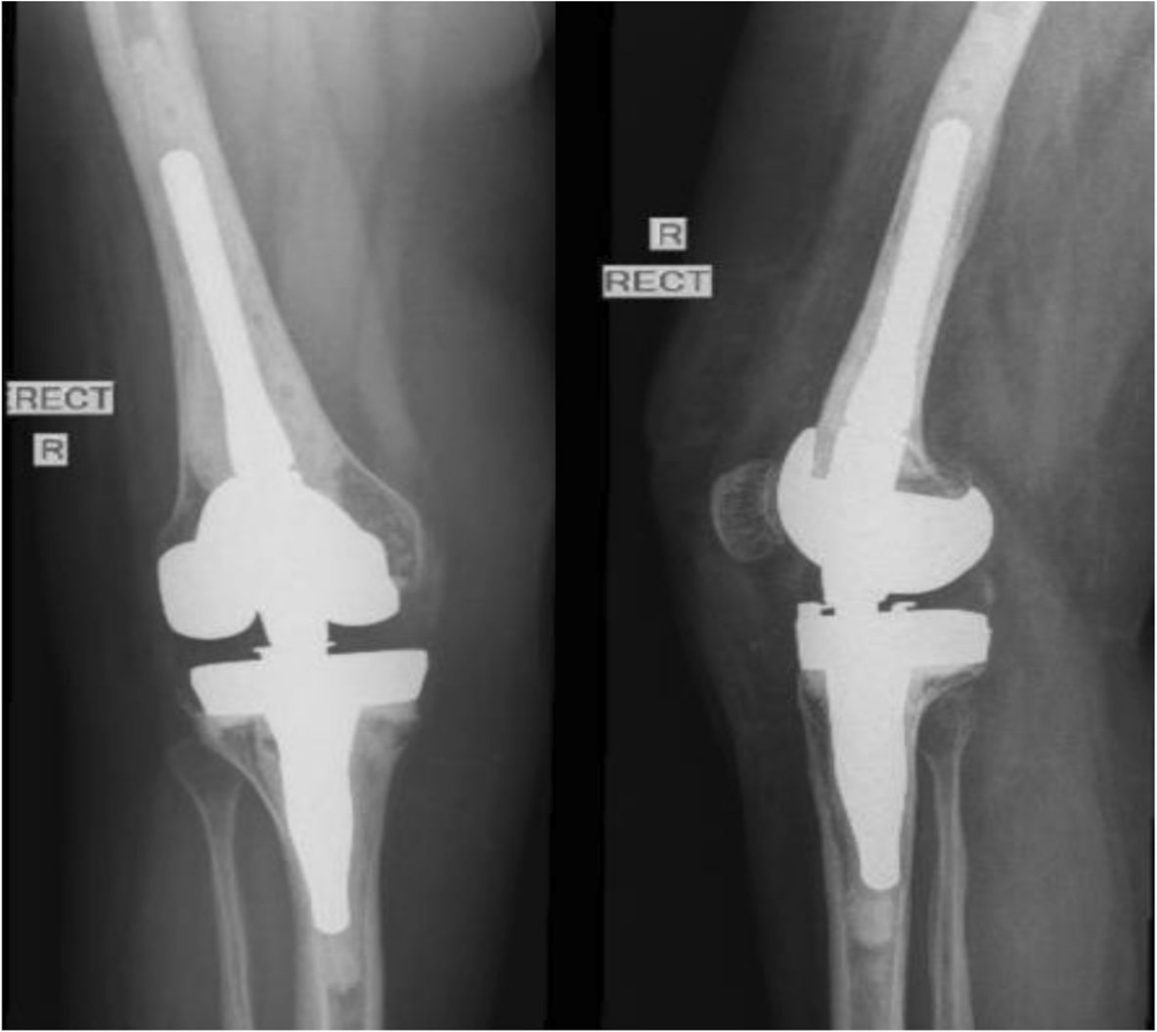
A postoperative AP and lateral radiograph of the knee demonstrating the cemented hinged prosthesis
